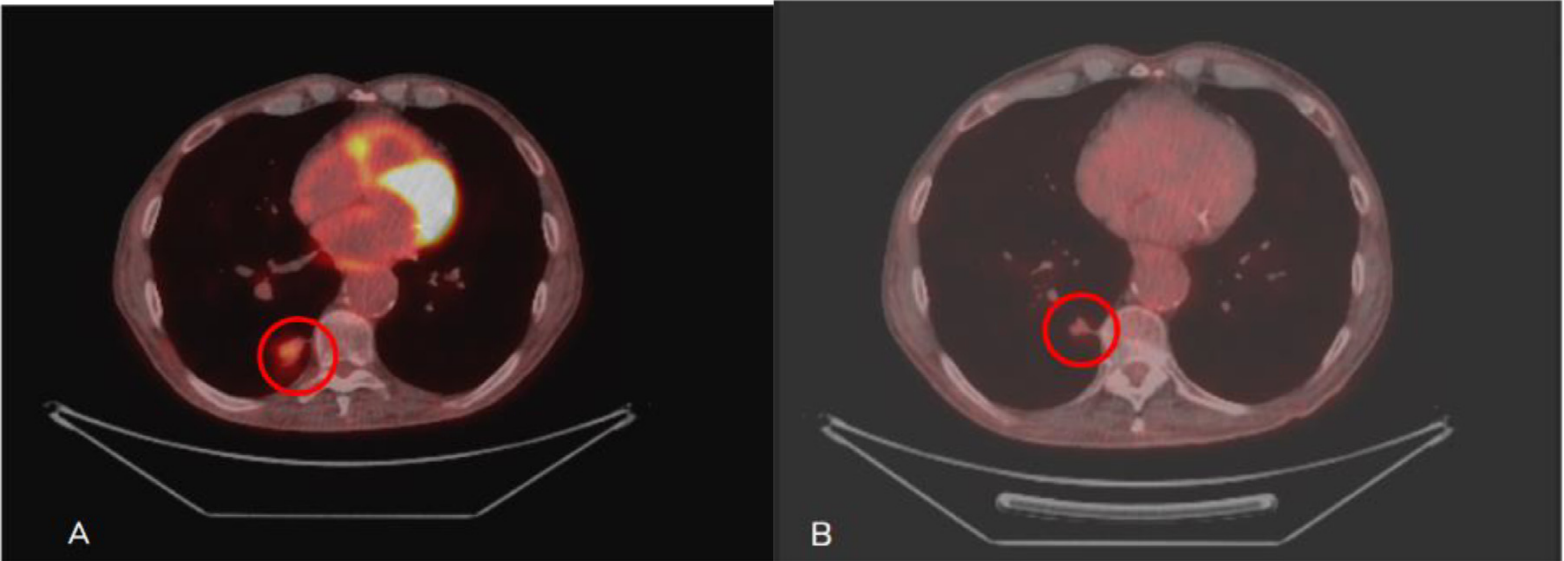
(A) PET/CT scan of the first patient at the time of diagnosis, revealing the lesion in the right lower lobe, with poor metabolic uptake (area into the circle). (B) PET/CT scan 6 months postcryoablation, showing no metabolic uptake at the primary tumor site (area into the circle).
